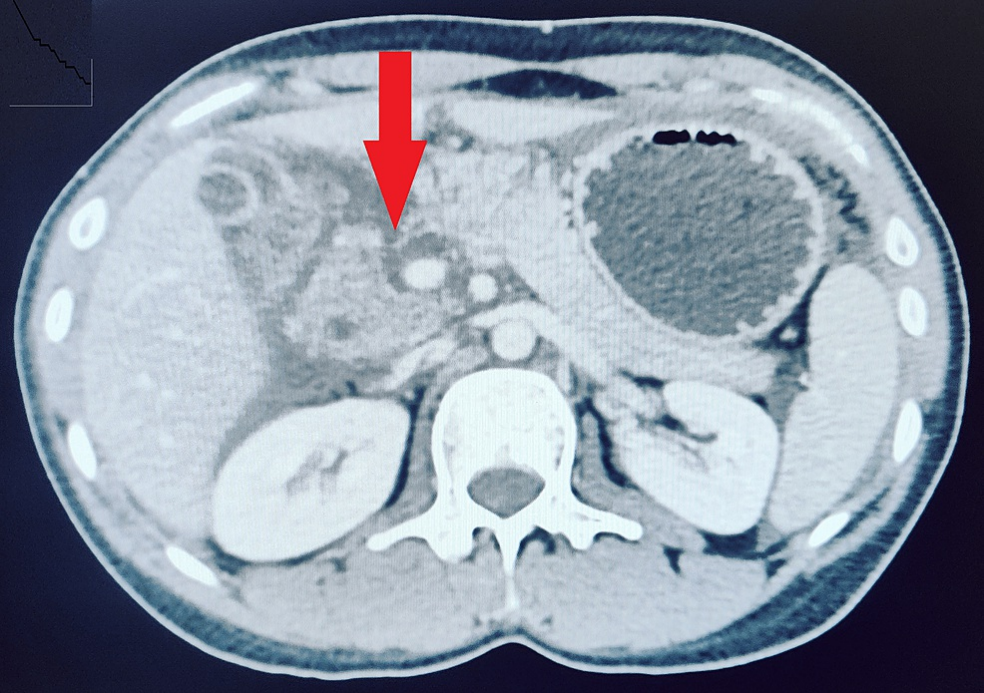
CT image showing pancreatic injury
